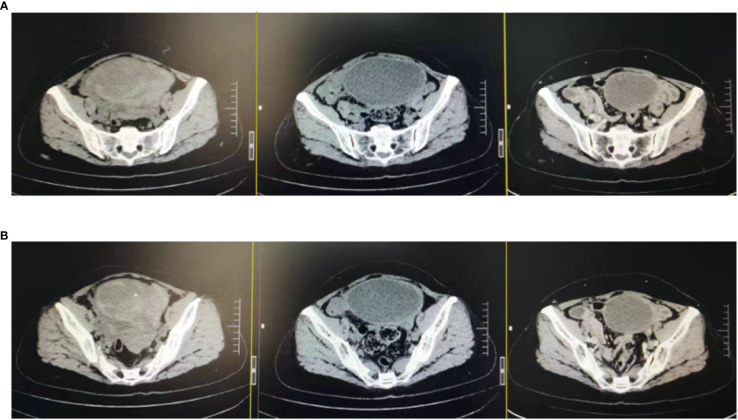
Changes in lesions: After the triple therapy, the CT showed a significantly smaller pelvic mass. (A) Pelvic mass before the triple therapy. (B) Pelvic mass after the triple therapy.
